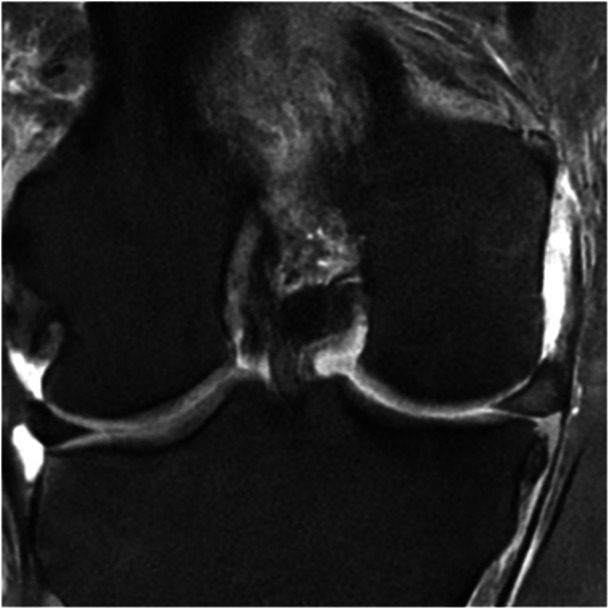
MRI coronal view of a right knee. Medial ME as well as signs of medial meniscal degeneration can be observed. A mild reduction of tibial chondral thickness is visible without subchondral changes nor osteophytes. ME, meniscal extrusion; MRI, magnetic resonance imaging.
